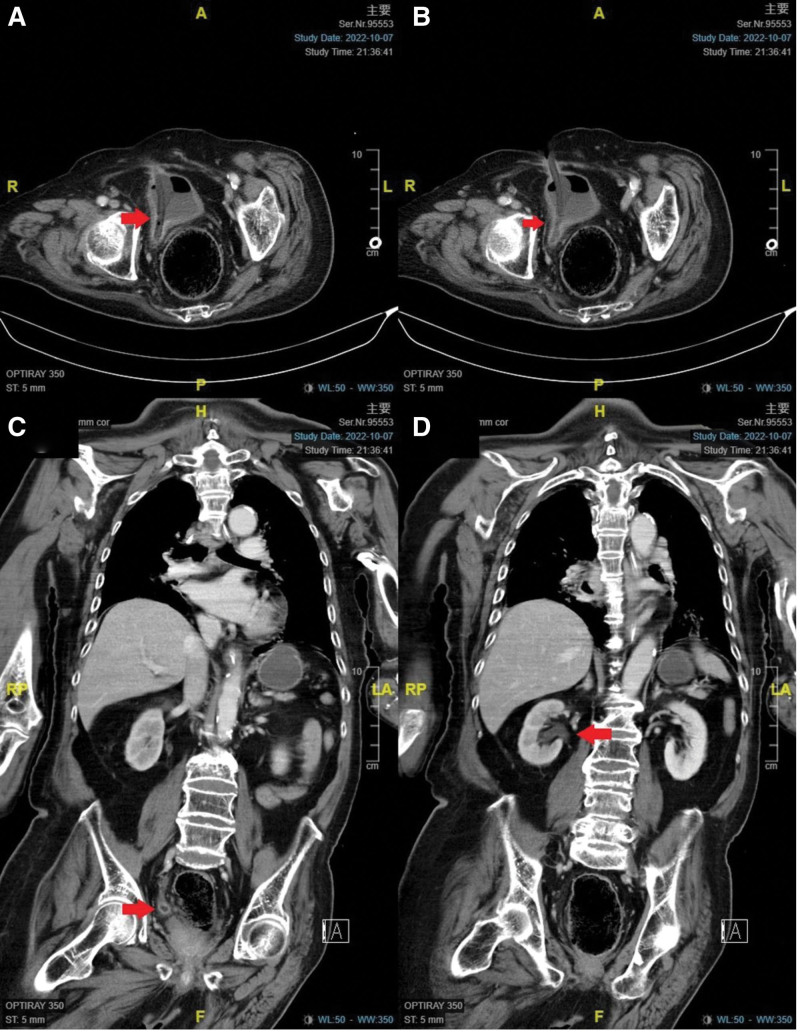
Abdomen and pelvic CT revealed (A–C) misplacement of the SPC tip (arrow) into the right ureter (D) right hydronephrosis (arrow) with perinephric fat stranding.
